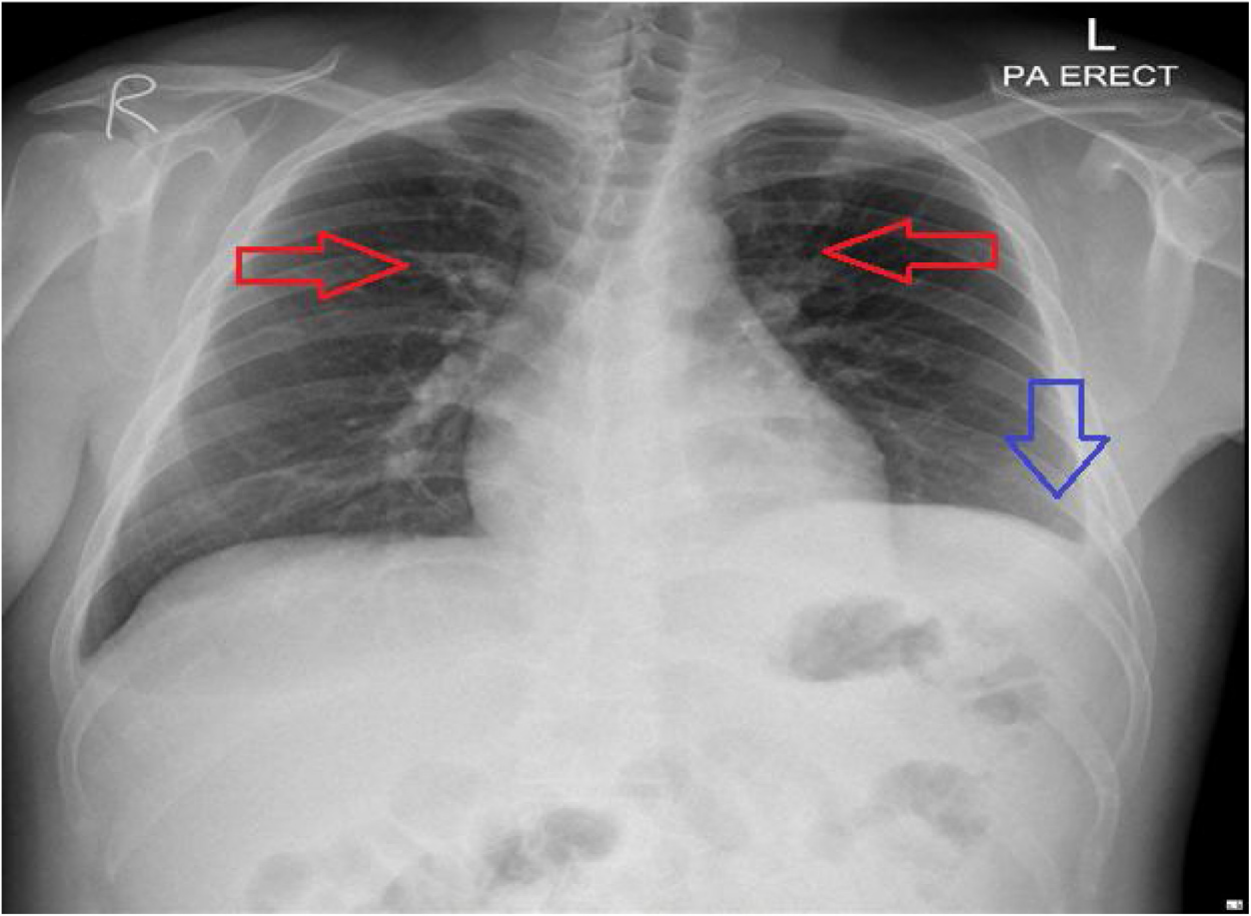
Chest X-ray upon his most recent admission. Mildly prominent hilar vascular markings identified (red arrows) with minimal blunting of the left costophrenic angle (blue arrows) and mild elevation of the left hemidiaphragm. But Cardio-mediastinal silhouette.
